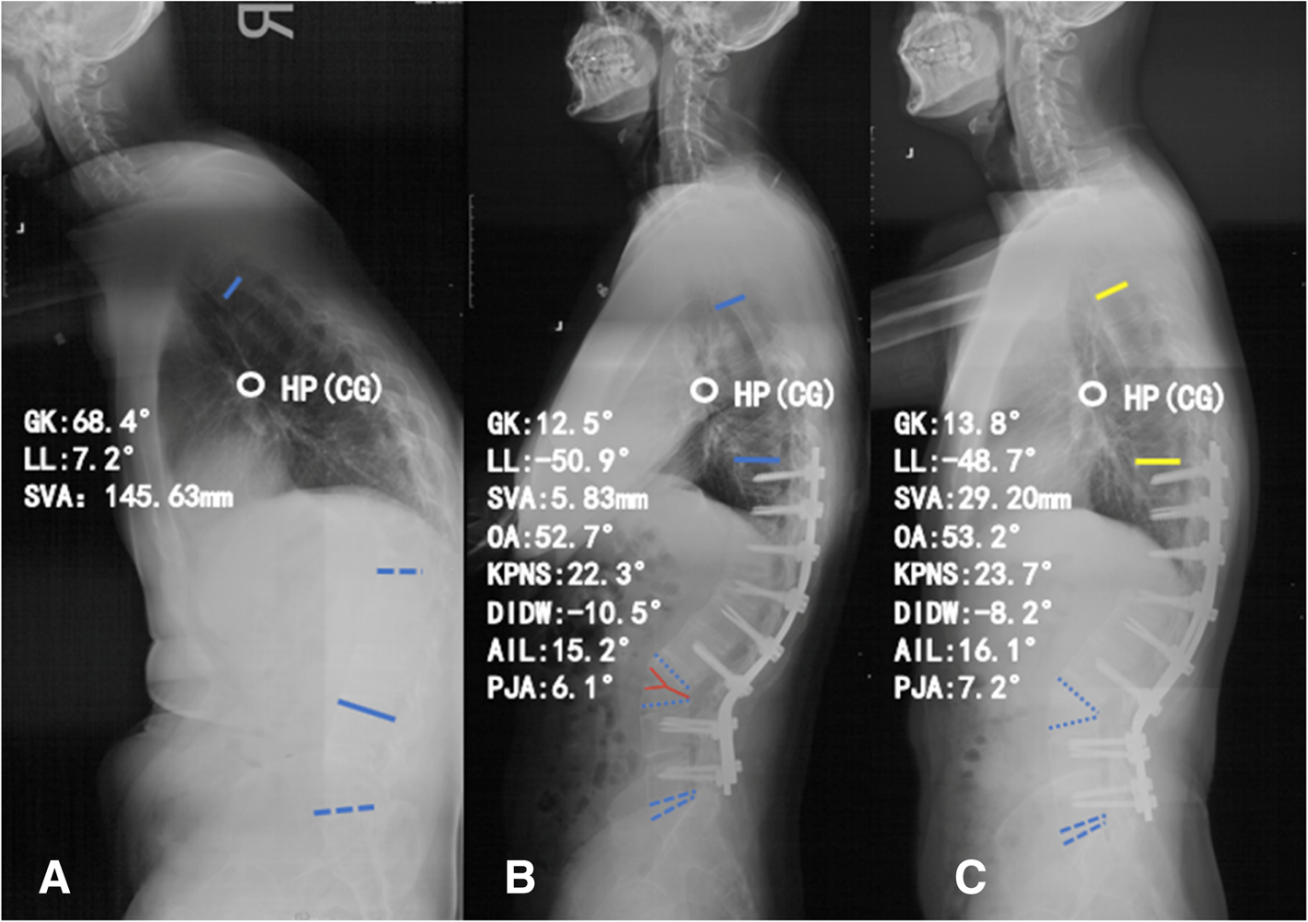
A 38-year-old male patient with thoracolumbar kyphosis secondary to ankylosing spondylitis. a Preoperative standing lateral radiograph. b Immediately after surgery with vertebral column decancellation (VCD) technique. c Lateral radiograph taken 32 months after surgery. AIL angle of instrumented levels, DIDW the sum of distal non-fused intervertebral disc wedging, GK global kyphosis, KPNS kyphotic angle of proximal non-fused segment involved in the global kyphosis, LL lumbar lordosis, OA osteotomized vertebra angle, PJA proximal junctional angle, SVA sagittal vertical axis, HP hilus pulmonis, CG the center of gravity
